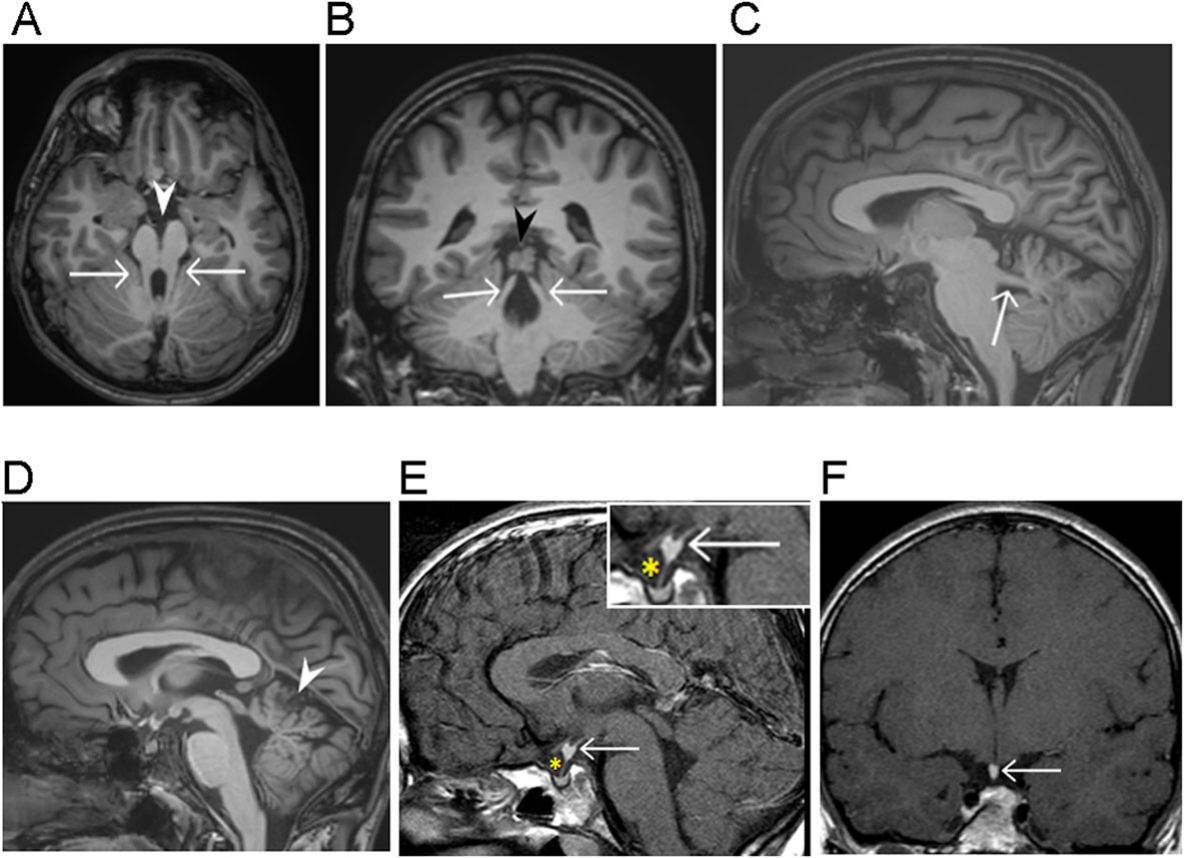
a-d Molar tooth sign in JBTS. Axial (a), coronal (b) and sagittal (c) T1-weighted images show large, thickened, elongated superior cerebellar peduncles (arrows). Note a deep interpeduncular fossa (white arrowhead) and the vermian cleft (black arrowhead). Midsagittal (d) T1-weighted image shows vermian hypoplasia (white arrowhead). e-f Ectopic posterior pituitary lobe. Postcontrast sagittal and coronal T1-weighted images show a posterior pituitary lobe (arrow) located at the level of the upper infundibulum. A very thin infundibulum is seen (asterisk) (magnified in the right upper corner of E).
